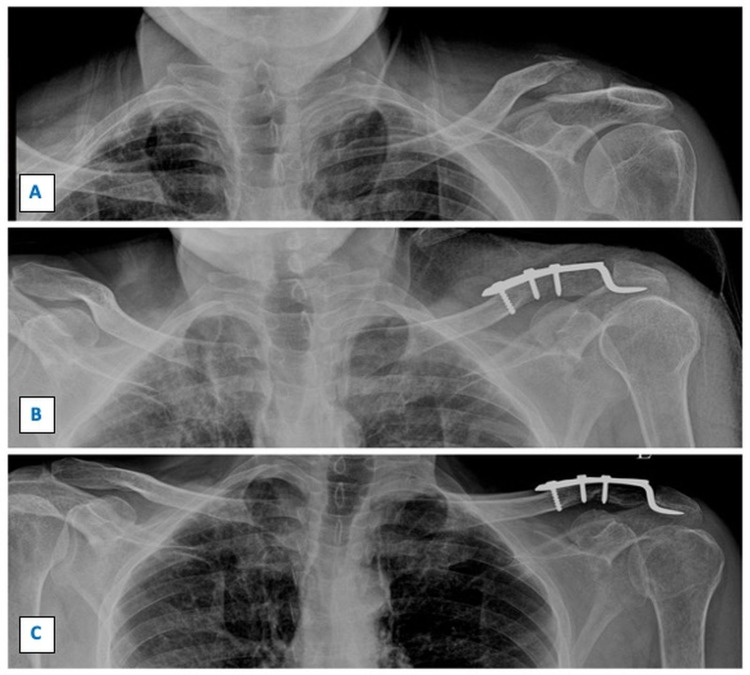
Sequential radiographs showing fracture of left lateral end clavicle managed by clavicular hook plate. Figure 2A shows the preoperative radiograph with displaced left lateral end clavicle fracture. Figure 2B shows immediate postoperative radiograph managed by clavicular hook plate. Figure 2C shows the radiograph at the one-year follow-up with the union of the fracture site.
